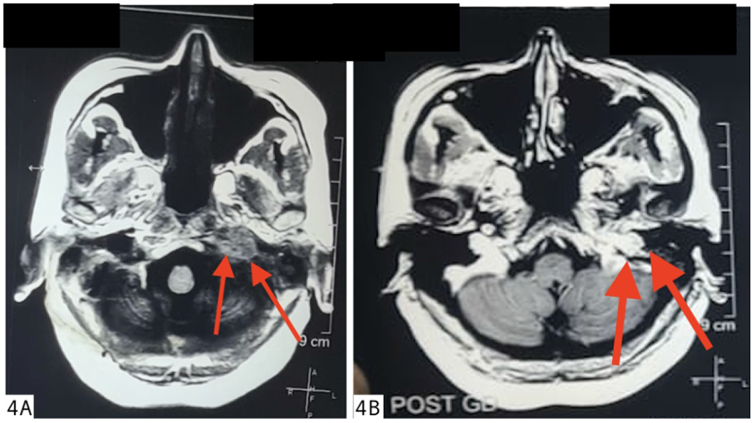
(A) and (B): Axial T1-weighted (left) and T1-weighted post-gadolinium (right) MRI images of the skull base. The red arrows indicate a soft tissue lesion on the left side, which appears low signal on T1W (left) and shows heterogeneous, avid enhancement post-gadolinium (right). The lesion causes lytic destruction of the left mastoid air cells and extends into the middle ear cavity.
